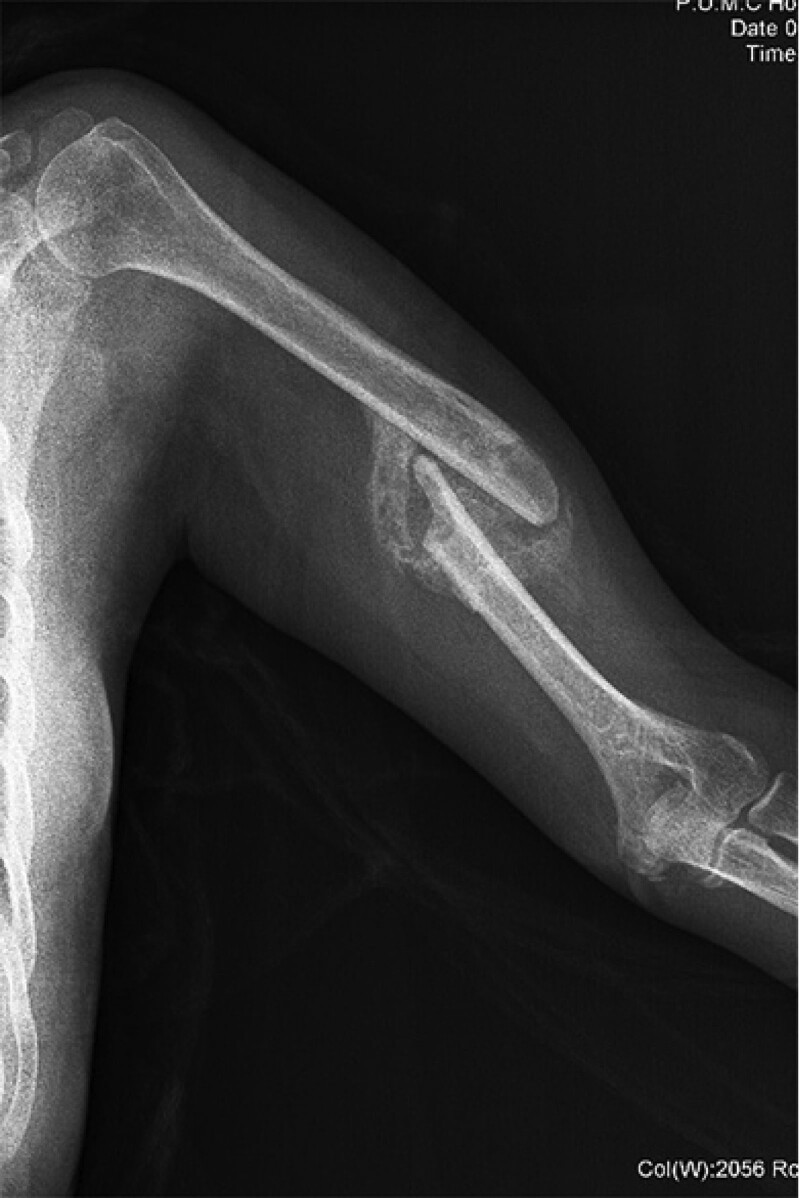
A radiograph of the left arm taken 4 mo after the accident reveals malunion of left humeral diaphysis with osseous bridge formation between displaced ends of fracture.
